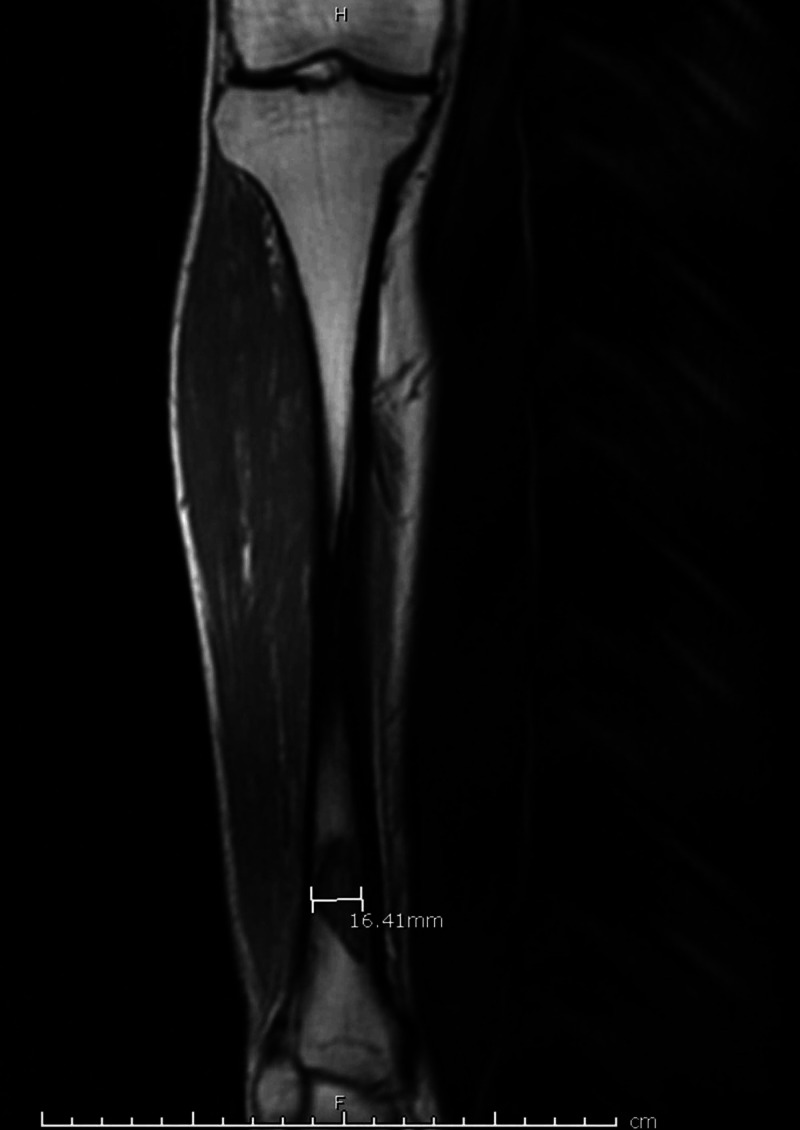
MRI of the right lower extremity (tibia and fibula) MRI shows osteomyelitis of the distal tibia with the formation of Brodie's abscess, which drains posteriorly into the soft tissues of the posterolateral tibia and fibula.
